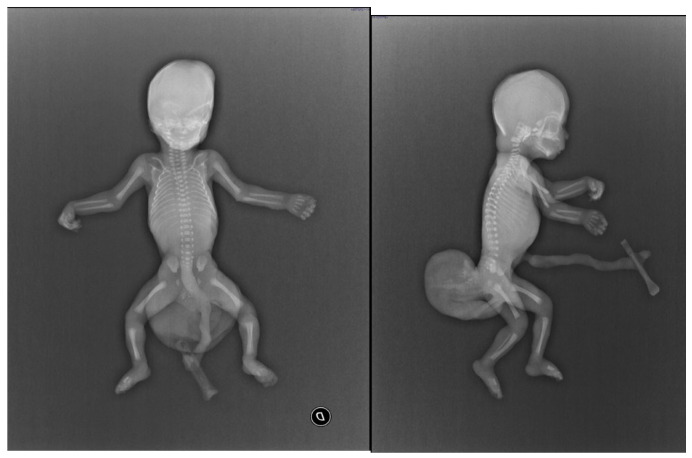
Immature sacrococcygeal teratoma. X-ray examination revealed a soft tissue mass with extra-foetal extension.
